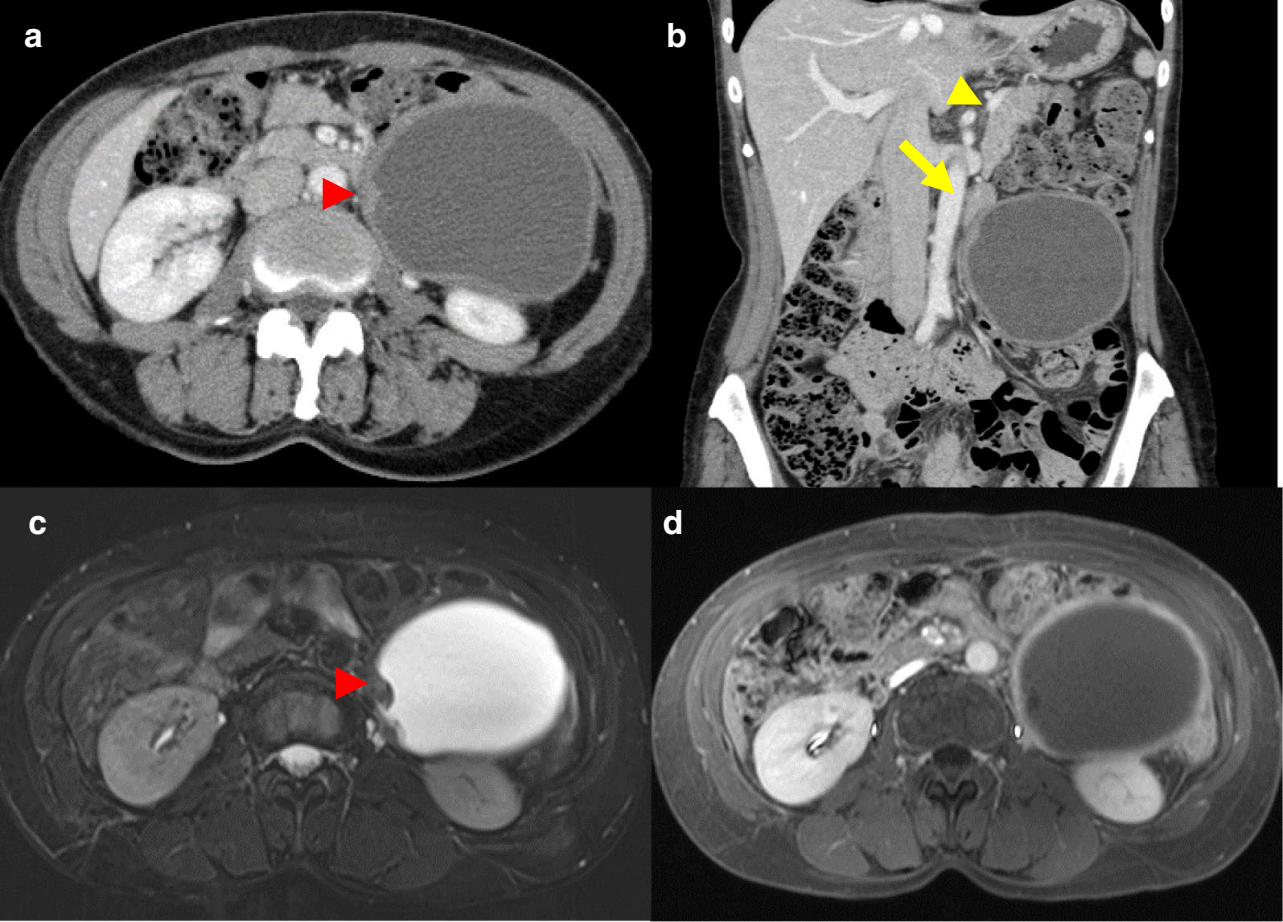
Preoperative abdominal contrast-enhanced computed tomography (CT) and magnetic resonance imaging (MRI) findings. a CT revealing a large unilocular cystic mass with three mural nodules (red arrow’s head) in the left retroperitoneal space on axial view. The boundary between the nodule and the wall was unclear in all three nodules. b CT demonstrating that the cystic mass was located close to the proximal side of the jejunum (yellow arrow) and the mass was separated from kidney and pancreas (yellow arrow’s head) on coronal view. c T1-weighted images revealing low signal intensity of the cystic wall, including mural nodules, and high signal intensity of the cyst component on axial view. In contrast to CT, mural nodules were clearly delineated at the rise. d T2-weighted images revealing high signal intensity of the cystic wall including mural nodules and low signal intensity of the cyst component on axial view
